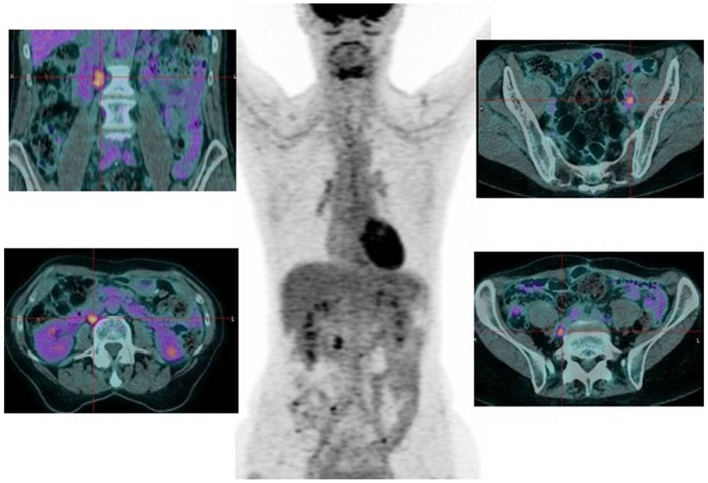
Seventy-two-year-old patient suspected of pelvic recurrence of ovarian cancer. The CT was negative and CA125 normal (12 U/mL for *N* < 35), but with increasing kinetic. PET/CT found nodal retroperitoneal and pelvic abnormalities.
